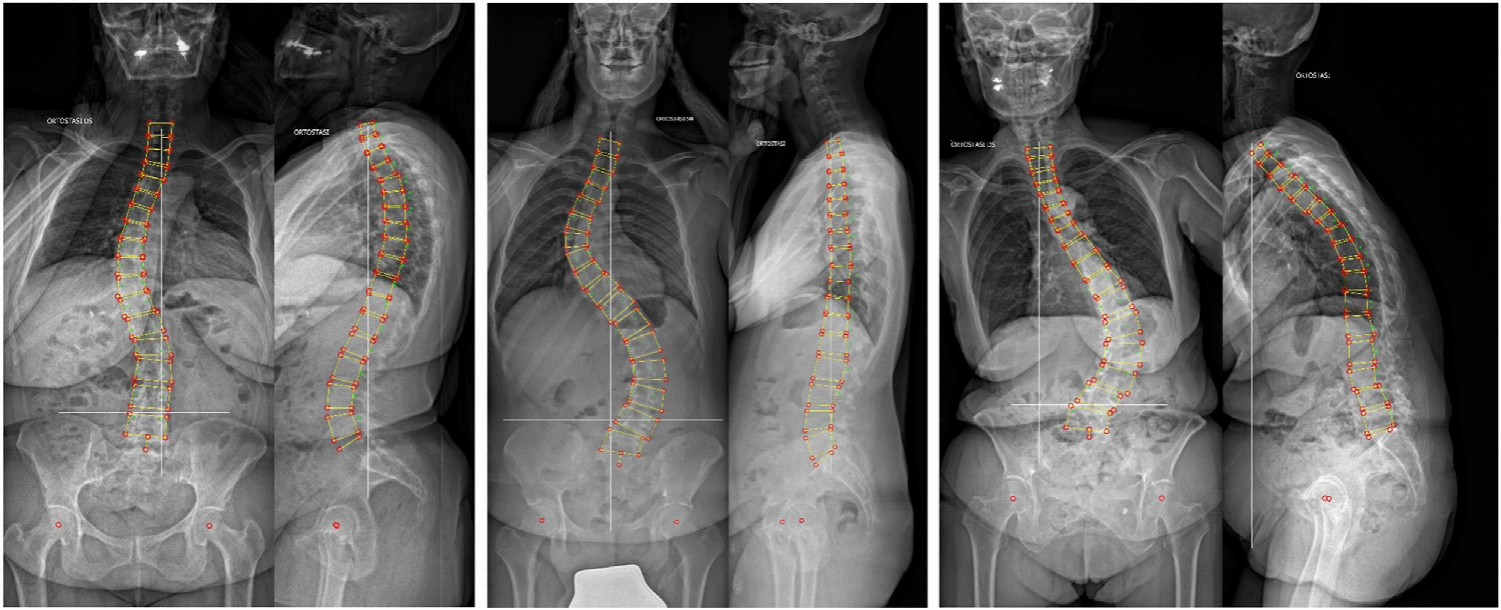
Three randomly selected representative cases of 3D reconstruction of the anatomy of the thoracolumbar spine based on biplanar radiographs of the trunk.
